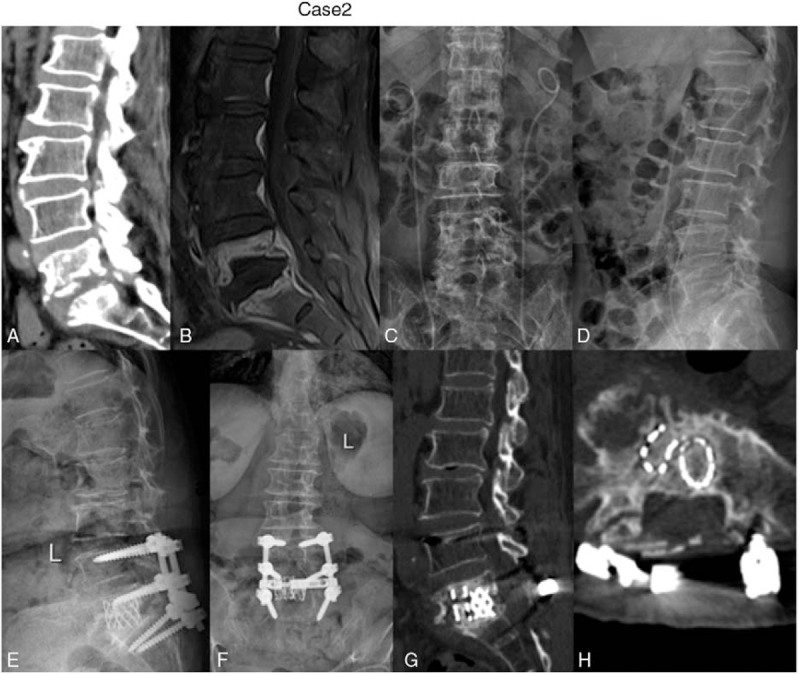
A 70-year-old female was diagnosed as having tuberculous spondylitis after an three months history of severe back pain. (A-D): x-ray, MRI, and CT showed the destruction of vertebral bodies of L5 and S1, (E–H) final follow-up radiographs showed good bone fusion.
